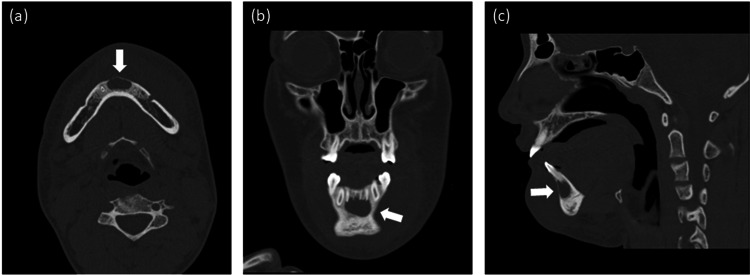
Computed tomography (a) Axial, (b) coronal, and (c) sagittal computed tomography images revealed the simple bone cyst in the mandibular bone.
